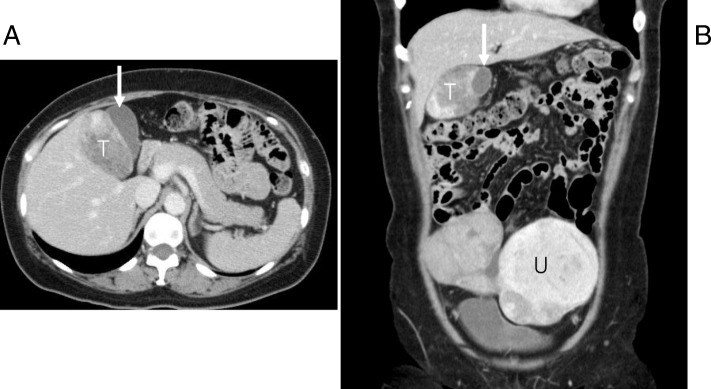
a, b Dynamic computed tomography demonstrated a hypodense tumor (T) measuring approximately 7.0 cm in diameter beside the gallbladder (white arrow) apart from the uterine fibrosis (U)
